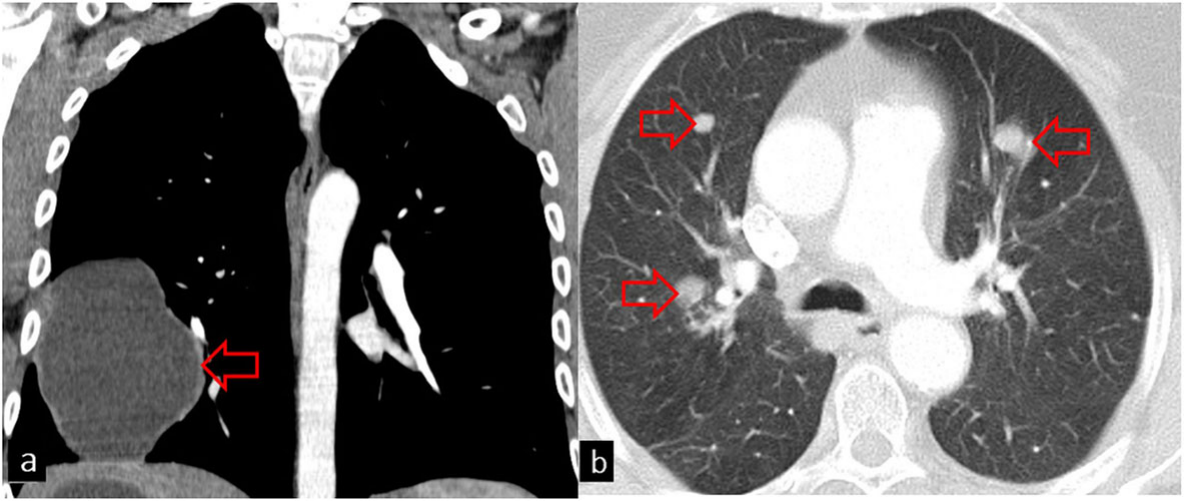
a Coronal chest CT image in the mediastinal window shows a giant (> 10 cm) uncomplicated hydatid cyst (red arrow). Measurement of HU values on CT shows low density (mean HU ± SD = − 1 ± 10) consistent with simple fluid. b On axial CT image, bilateral hydatid cysts are seen (red arrows)
